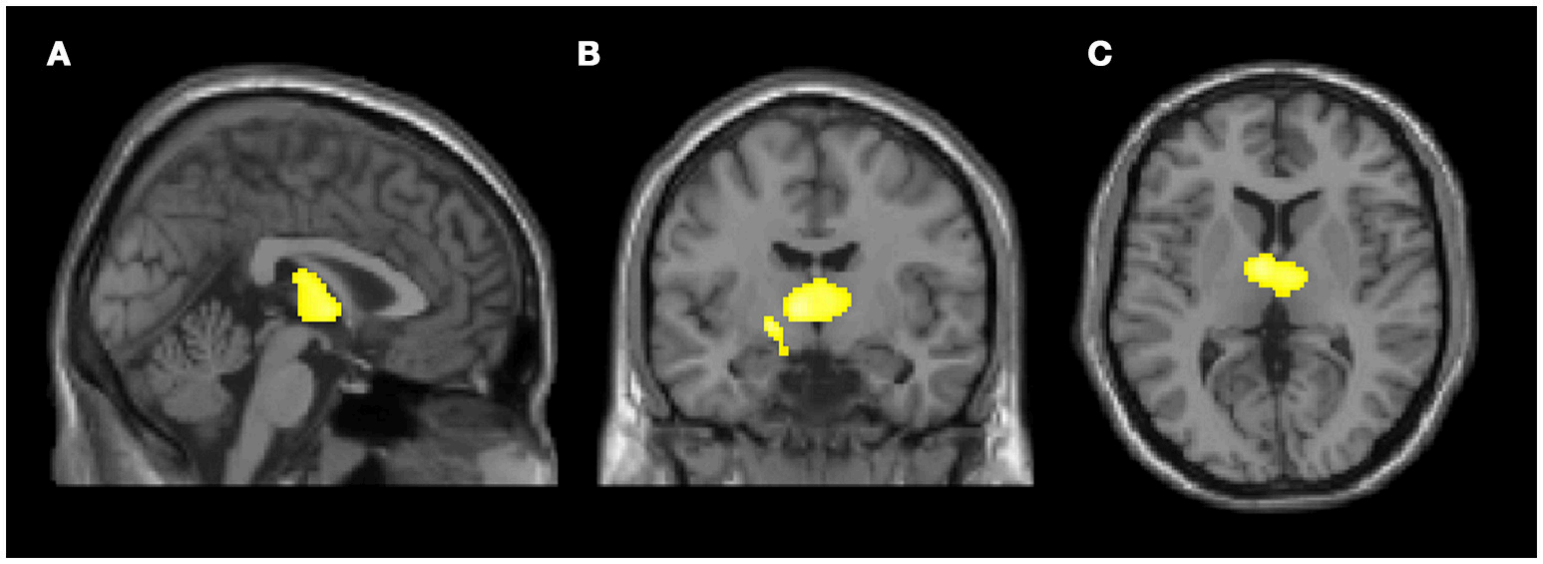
Clusters of gray matter loss in Fabry disease (FD) patients compared to healthy controls (HC). Results are displayed for *p* < 0.05 FWE-corrected at cluster level, and superimposed for anatomical reference to multi-planar reconstruction of a single subject T1-weighted volume in the standard Montreal Neurological Institute space (A) sagittal, (B) coronal, (C) axial. No region of increased gray matter volume was present in FD patients compared to HC.
